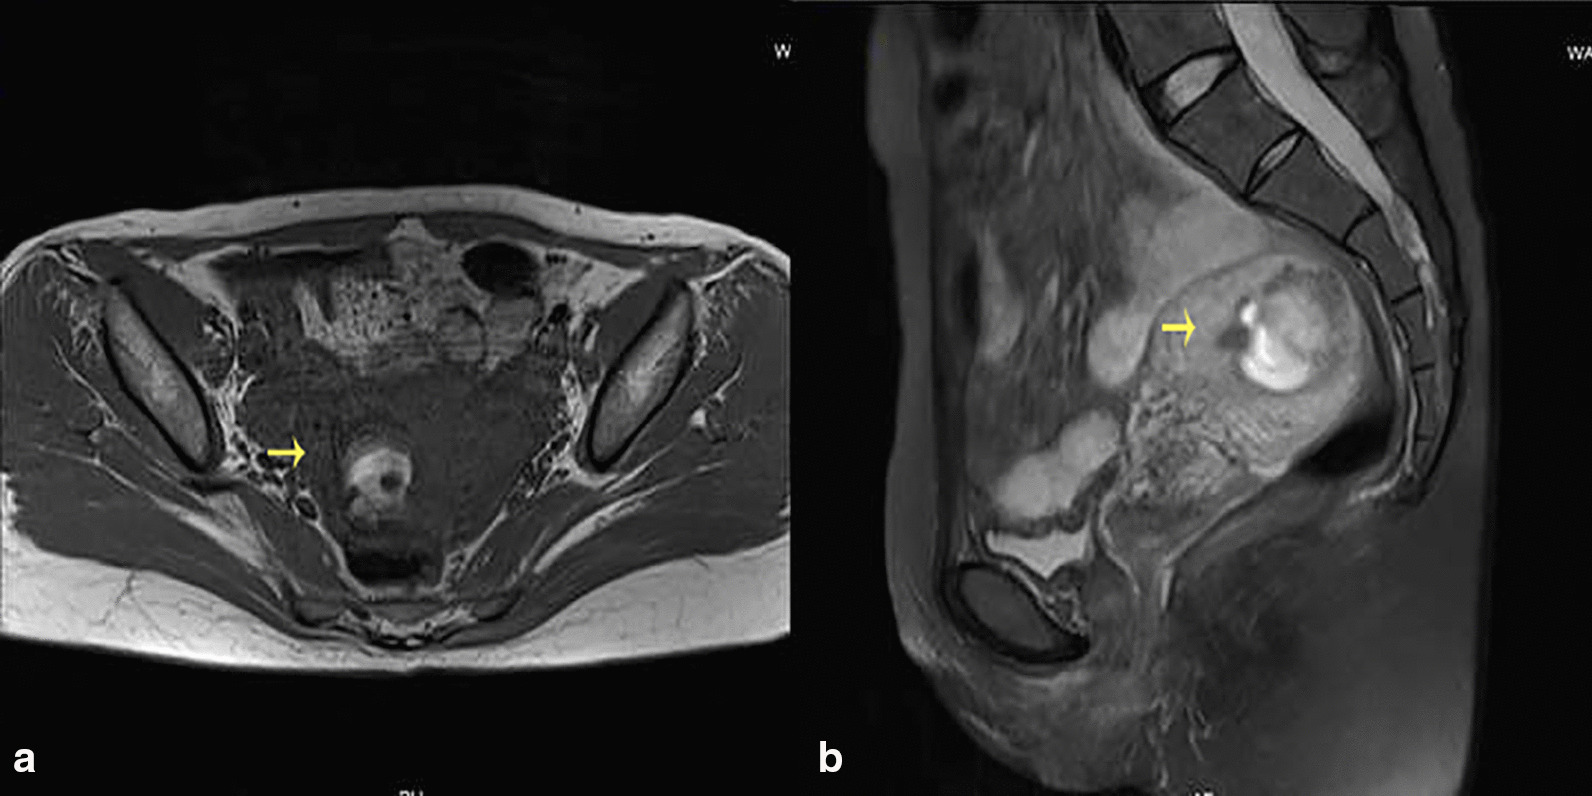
MRI image of case 1. Postoperative image of artificial abortion, mixed signal mass in the right harem of uterus, suggesting the possibility of residual. The arrow points to the gestation tissue
